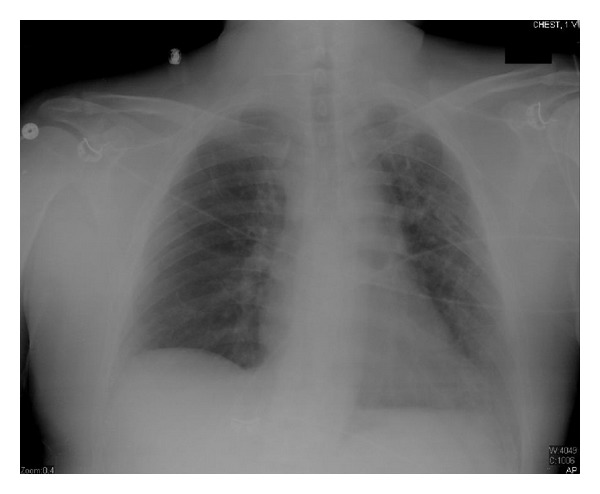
Chest X-ray day 31 after chemotherapy (ANC was 6700 cell/*μ*L).
